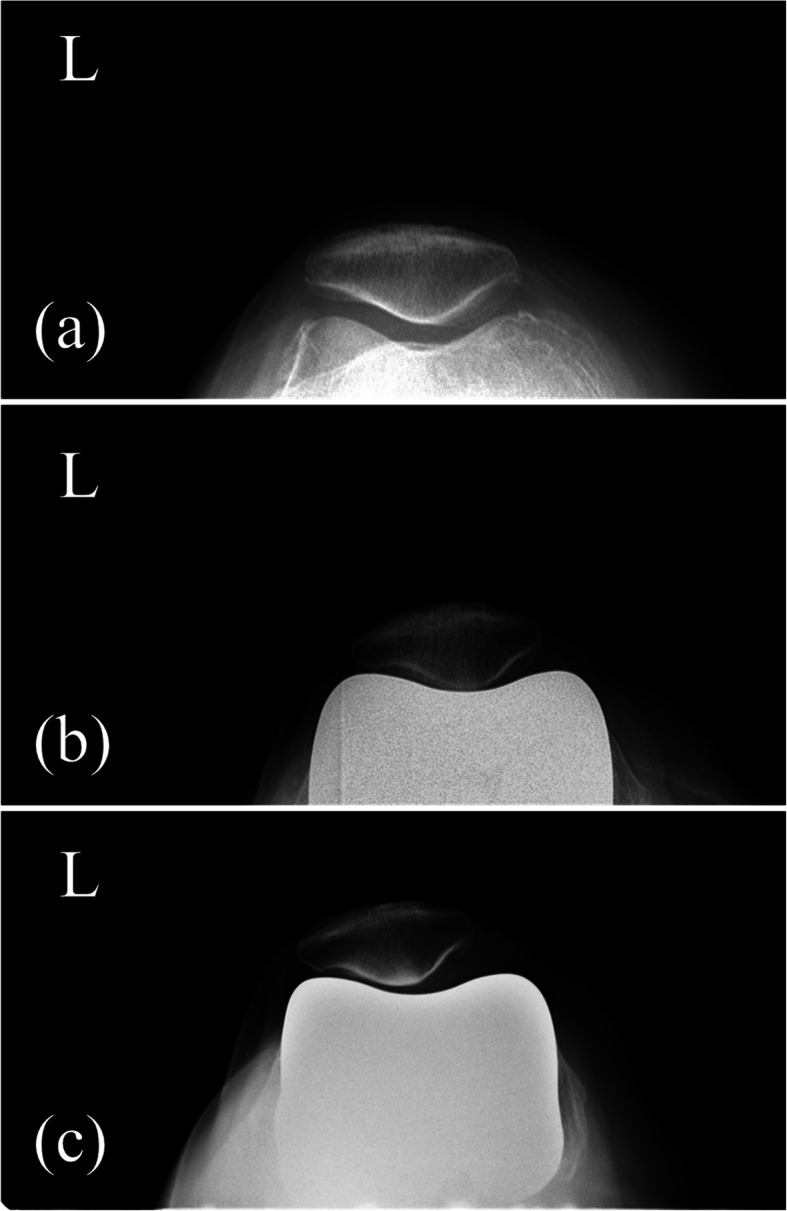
Progression of osteosclerosis. Progressive osteosclerosis was observed in the radiograph taken before the surgery (a), 1 year after the surgery (b), and 3 years after the surgery (c)
